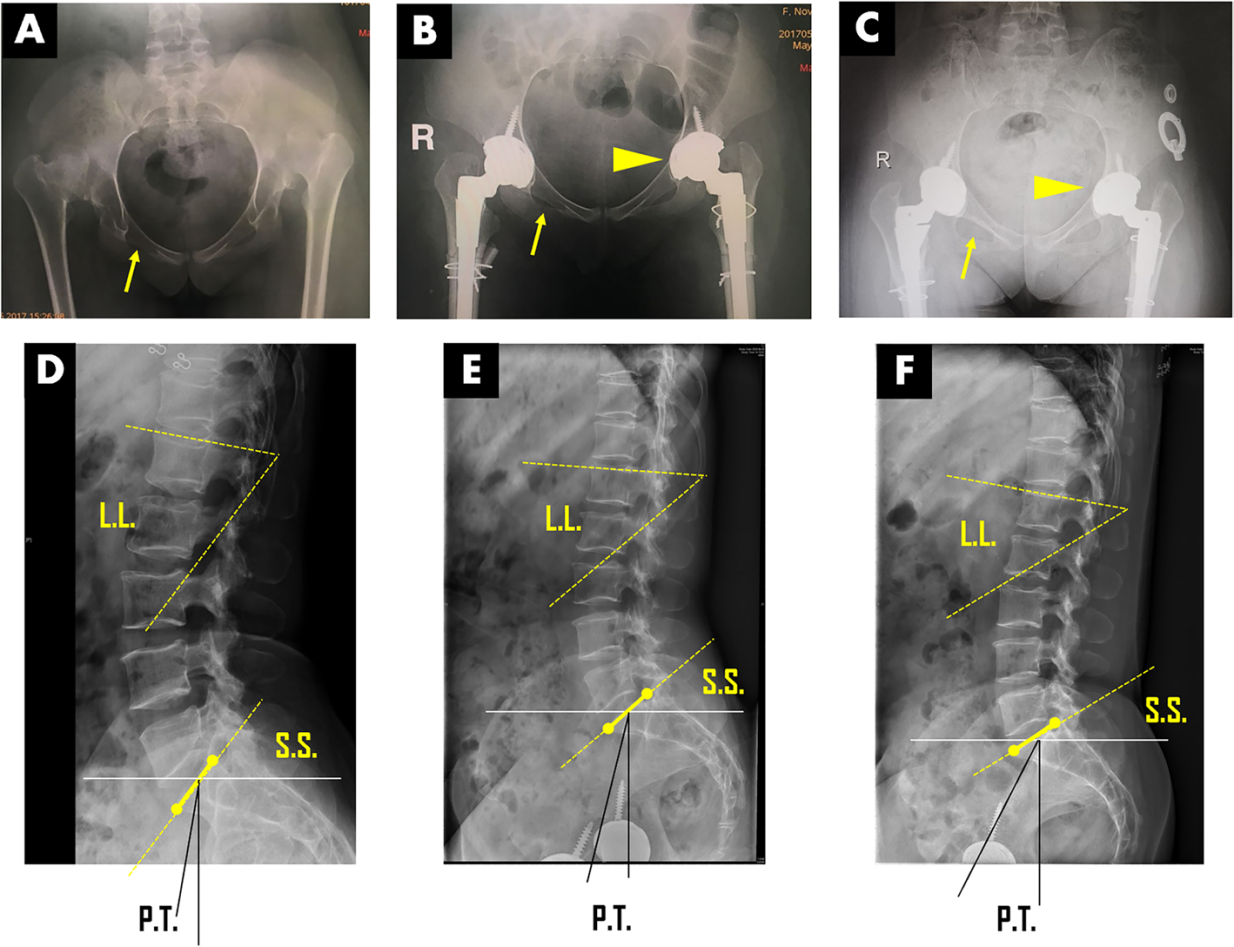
Specific temporal and spatial adaption observed from anteroposterior and lateral views of lumbar-pelvis X-ray in a bilateral high-dislocated DDH patient after DAA. A, D Preoperative anteroposterior pelvis X-ray demonstrated an extremely anteverted pelvis with opened inlet and closed obturator foramen (yellow arrow). The lateral X-ray of lumbar spine showed 64° of LL, 53° of SS, and 9° of PT. B, E Hip-spine adaption at the 6^th^ month after surgery, the anteroposterior pelvis X-ray showed slightly retroverted pelvis with opened obturator foramen (yellow triangle). And the inclination and anteversion angles of the acetabular component were 45° and 11° in right hip, and 38° and 9° in left hip. The lateral X-ray of lumbar spine showed 43° of LL, 40° of SS, and 14° of PT. C, F Hip-spine adaption at the 10^th^ month after surgery, the inclination and anteversion angles of the acetabular component transformed as 48° and 16° in the right, and 40° and 15° in the left (yellow triangle). The lateral X-ray of lumbar spine showed remodeled hip-spine complex with 42° of LL, 31° of SS, and 27° of PT. Please be noted the significant morphological change of obturator foramen (yellow arrow)
